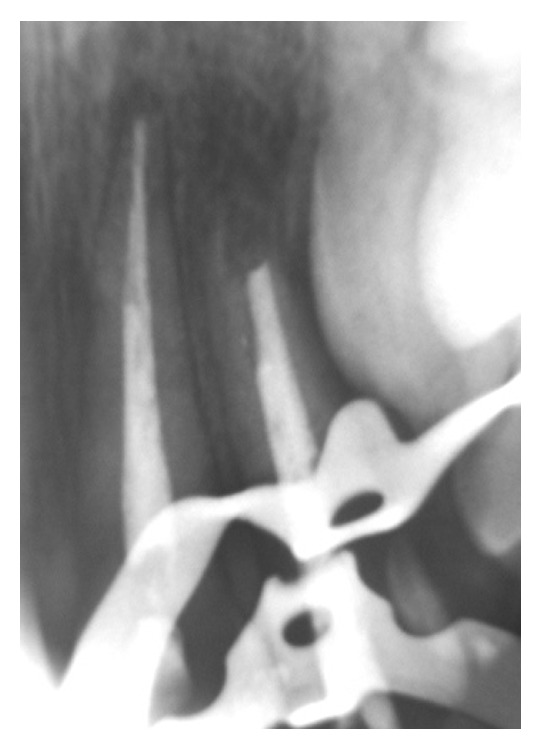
Periapical radiograph after 1 year of starting treatment showing the apical barrier formation after 8 months (seventh session; March 1999).
